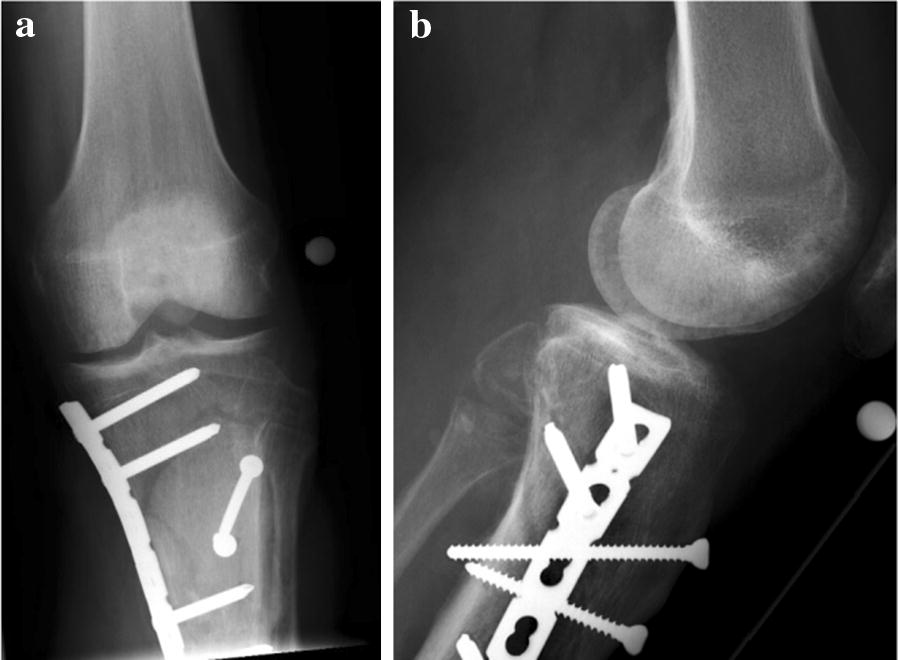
X-rays at first admission (AO type 41-A2, Anderson Gustilo IIIb, Tscherne/Oestern type III open fracture) after ORIF in Bulgaria. a X-ray a.p. view and b X-ray lateral view
